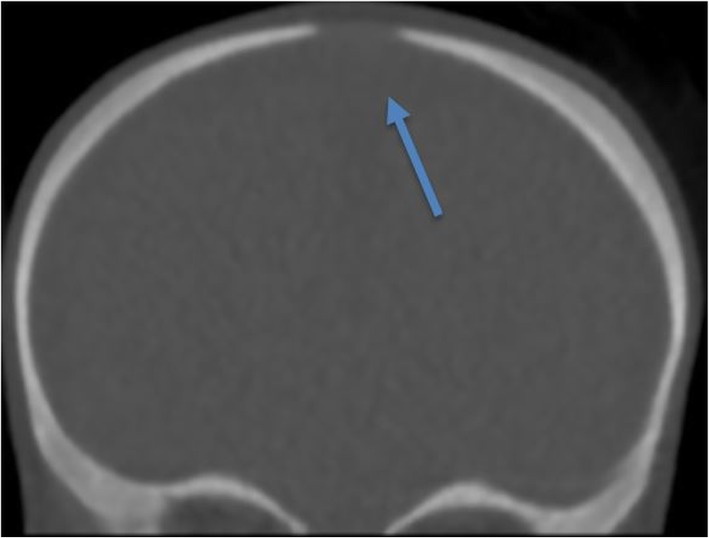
Coronal head CT image (craniosynostosis protocol) of a 4-month-old male (7 kg) acquired at 1.5 mGy. Anterior fontanelle without evidence of craniosynostosis (arrow) and overall image quality scored optimal by all readers
